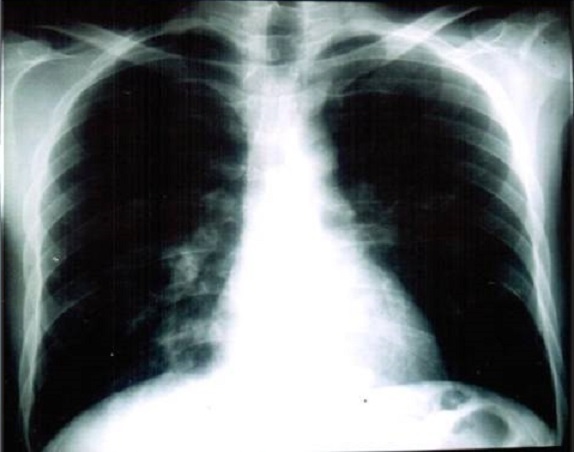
Radiographie thoracique montrant une image de caverne séquellaire parahilaire droite
